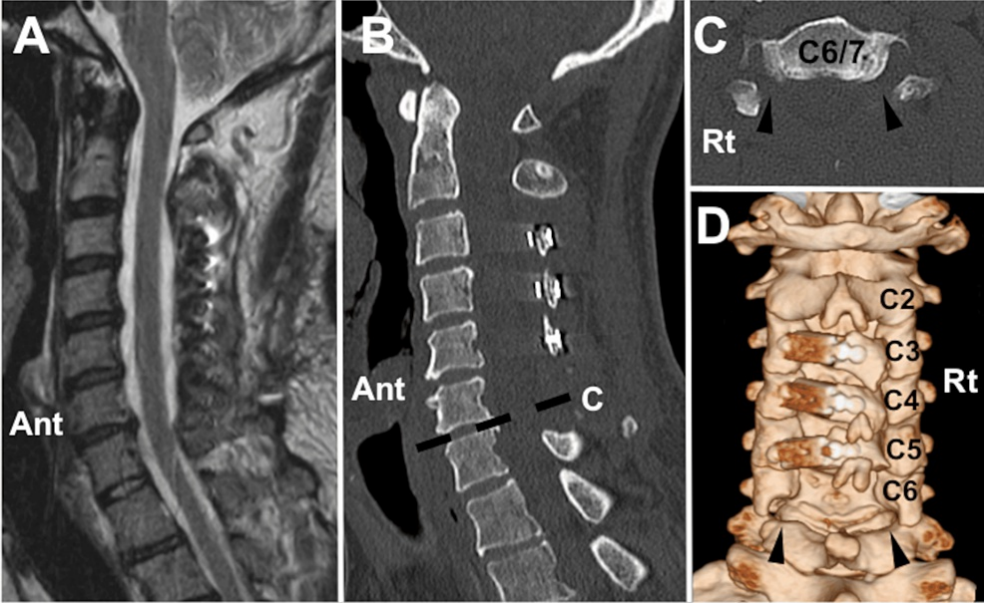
Radiographic images after the operation (case 2). Cervical canal stenosis was resolved after the operation (A: sagittal magnetic resonance image, B: sagittal computed tomography image). Posterior decompression is achieved after laminectomy and foraminotomy in the bilateral C6/7 levels (C). Left laminoplasty from C3 to C5 is combined with laminectomy of C6 and foraminotomy of the bilateral C6/7 levels (Ant: anterior, Rt: right)
